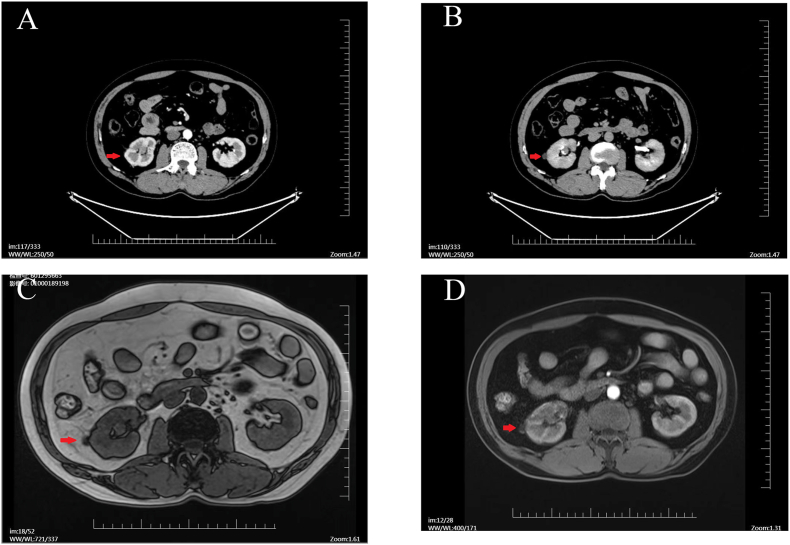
Pre-operative imaging data. A–B: CT images. C–D: MRI images.
